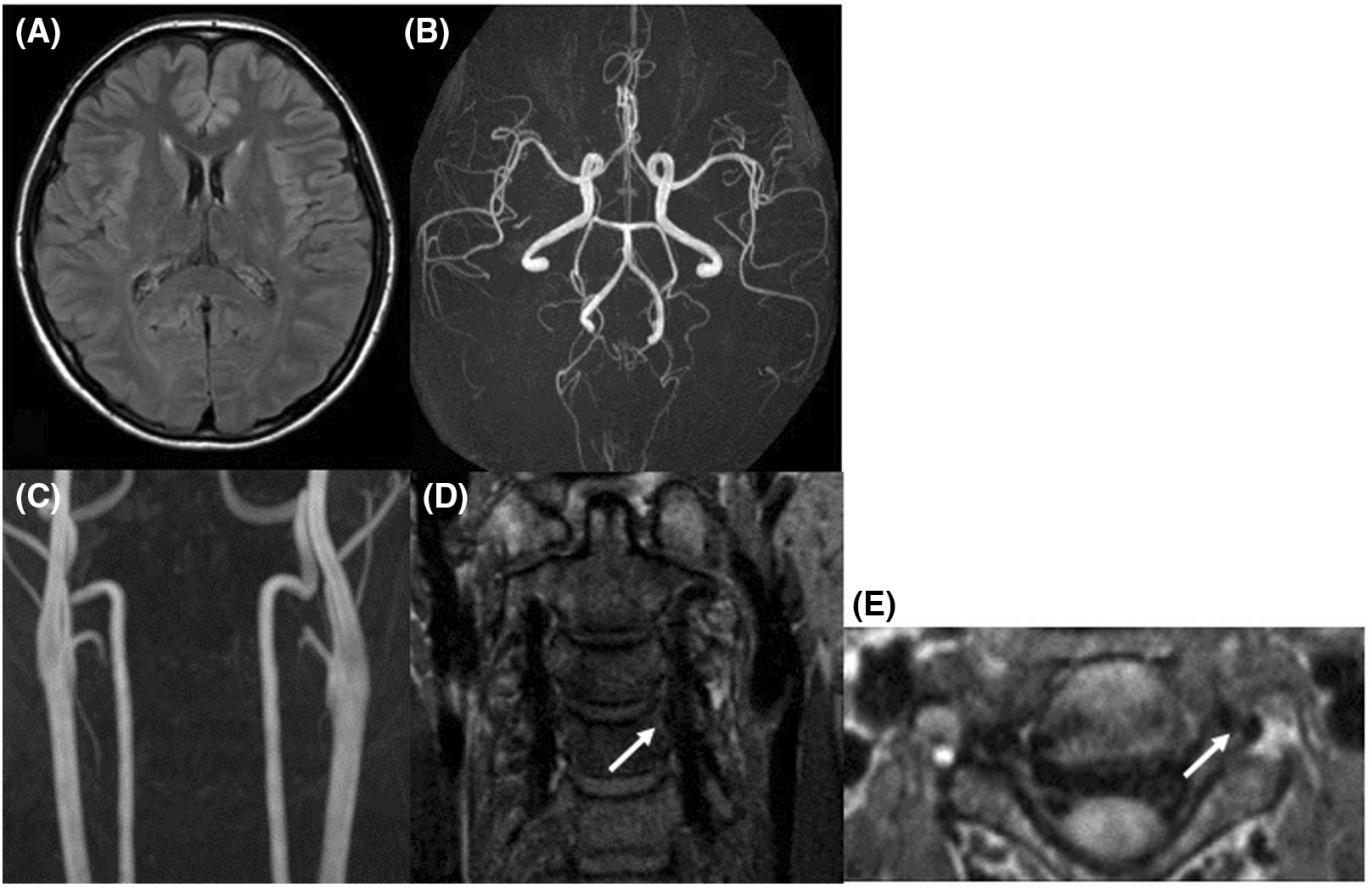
Neuroradiological findings 3 months after onset. (A) FLAIR images revealed the disappearance of initial abnormal lesions, and (B, C) MRA findings were normalized. MSDE coronal (D) and axial (E) showed the disappearance of a periluminal rim (white arrow). Based on the changes in findings over time, we diagnosed this lesion as vertebral artery dissection
